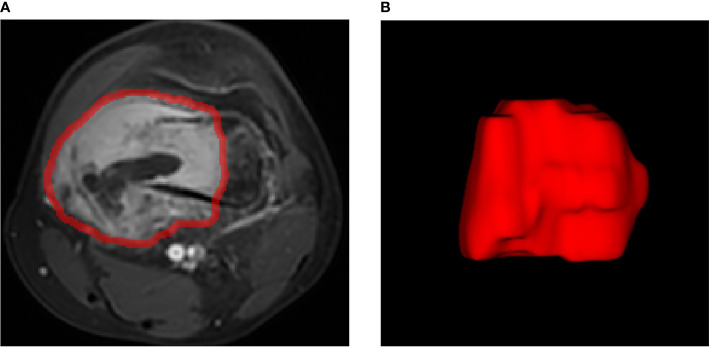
Example image for osteosarcoma contouring. (A) Outline of regions of interest on one slice of axial T1-weighted MR image of the strongest enhanced phase. (B) Volume rendering.
